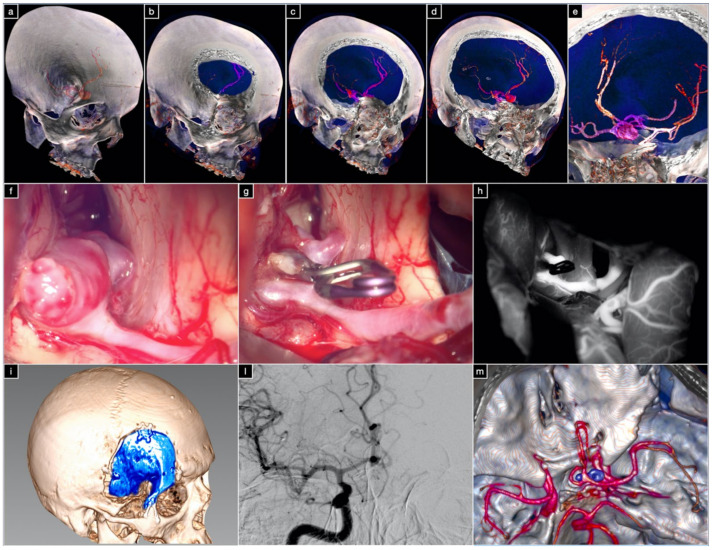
(a–e) Three-dimensional (3D) volume-rendered preoperative CT angiography. Intraoperative pictures taken before (f) and after (g) clipping of the aneurysm. (h) Postclipping indocyanine green videoangiography. (i) Three-dimensional (3D) volume-rendered postoperative CT scan showing the orbitopterional approach. (l) Digital subtraction angiography of the right internal carotid artery in anterior–posterior projection. (m) Postoperative 3D volume-rendered CT angiography.
